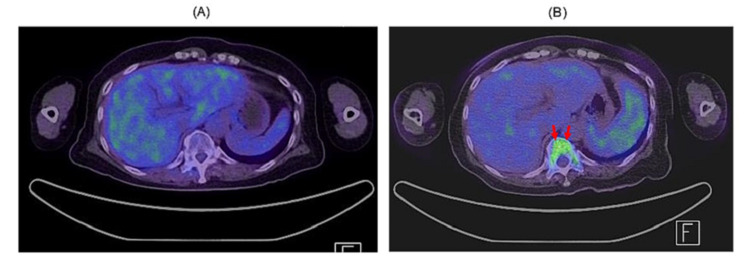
Positron emission tomography-computed tomography findings (A; six years previously, B; current study) (A) No abnormal accumulation was observed. (B) Splenomegaly was observed, and the spleen and vertebral body (bone marrow, red arrow) both had more accumulation (a maximal standardized uptake value ranging from 3.5-5.0) than the liver.
